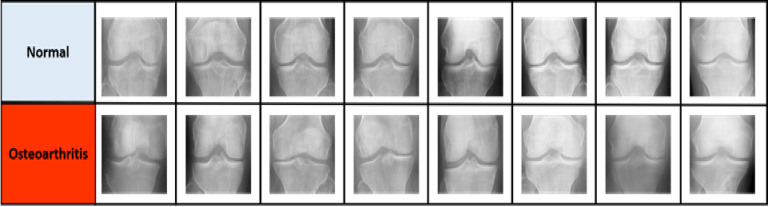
Samples of knee X-rays from the utilized dataset^50^.
